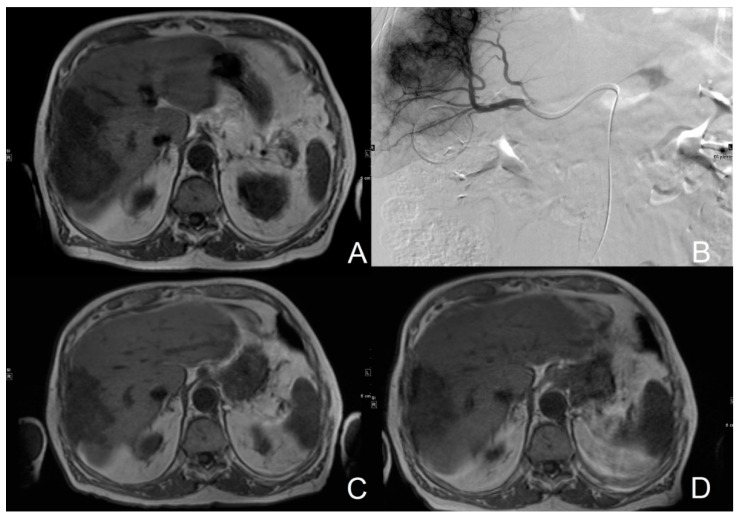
T1-weighted MR images of a 78-year old patient with non-resectable liver metastases of colorectal carcinoma prior to palliative cTACE (A). Angiogram during cTACE procedure shows liver metastases (B). Repetitive cTACE resulted in stabilization of disease (C). 20 months after initial chemoembolization, imaging still showed stabilization of disease (D).
